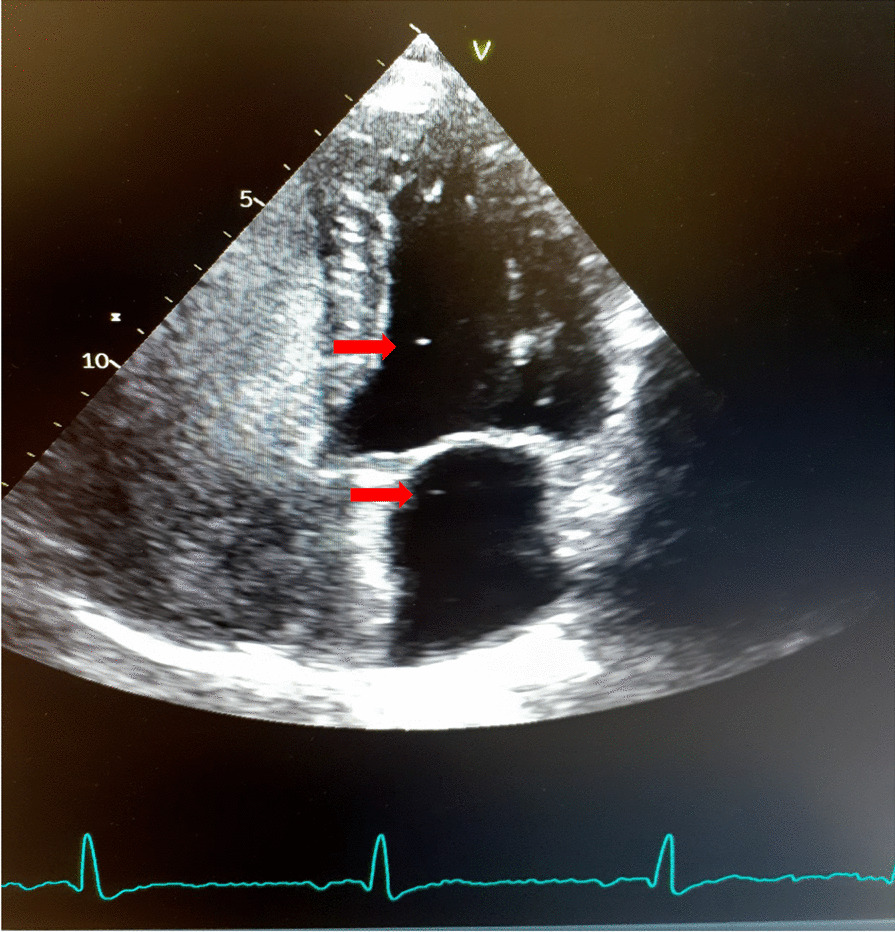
Echocardiogram (May 2019) that shows several bubbles in the left heart, eight cardiac cycles after the injection of agitated saline. This indicated that pulmonary arteriovenous malformations are likely present. Bubbles highlighted by red arrows
